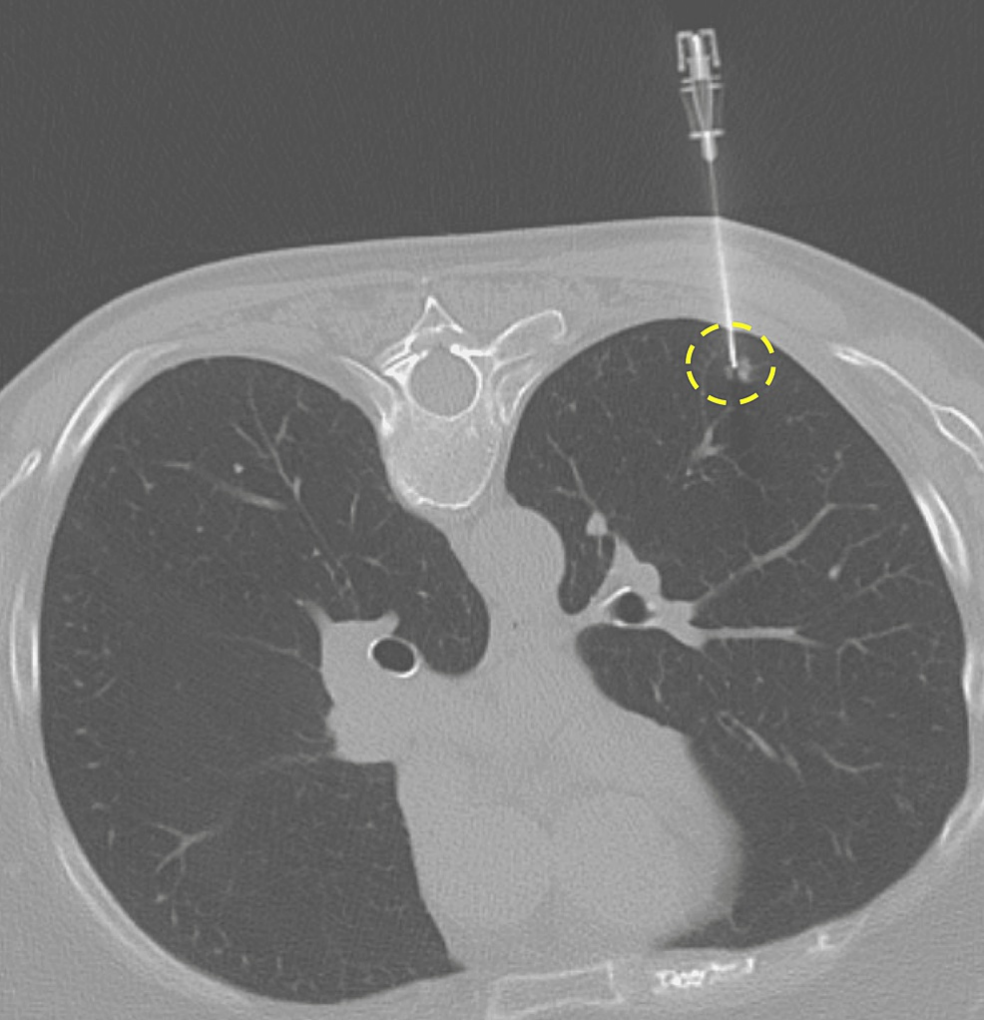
Unenhanced CT of the chest with lung biopsy algorithm The axial image through the level of the left lower lobe nodule (perforated yellow circle) with a biopsy trocar needle in place. Note: patient in prone position.
